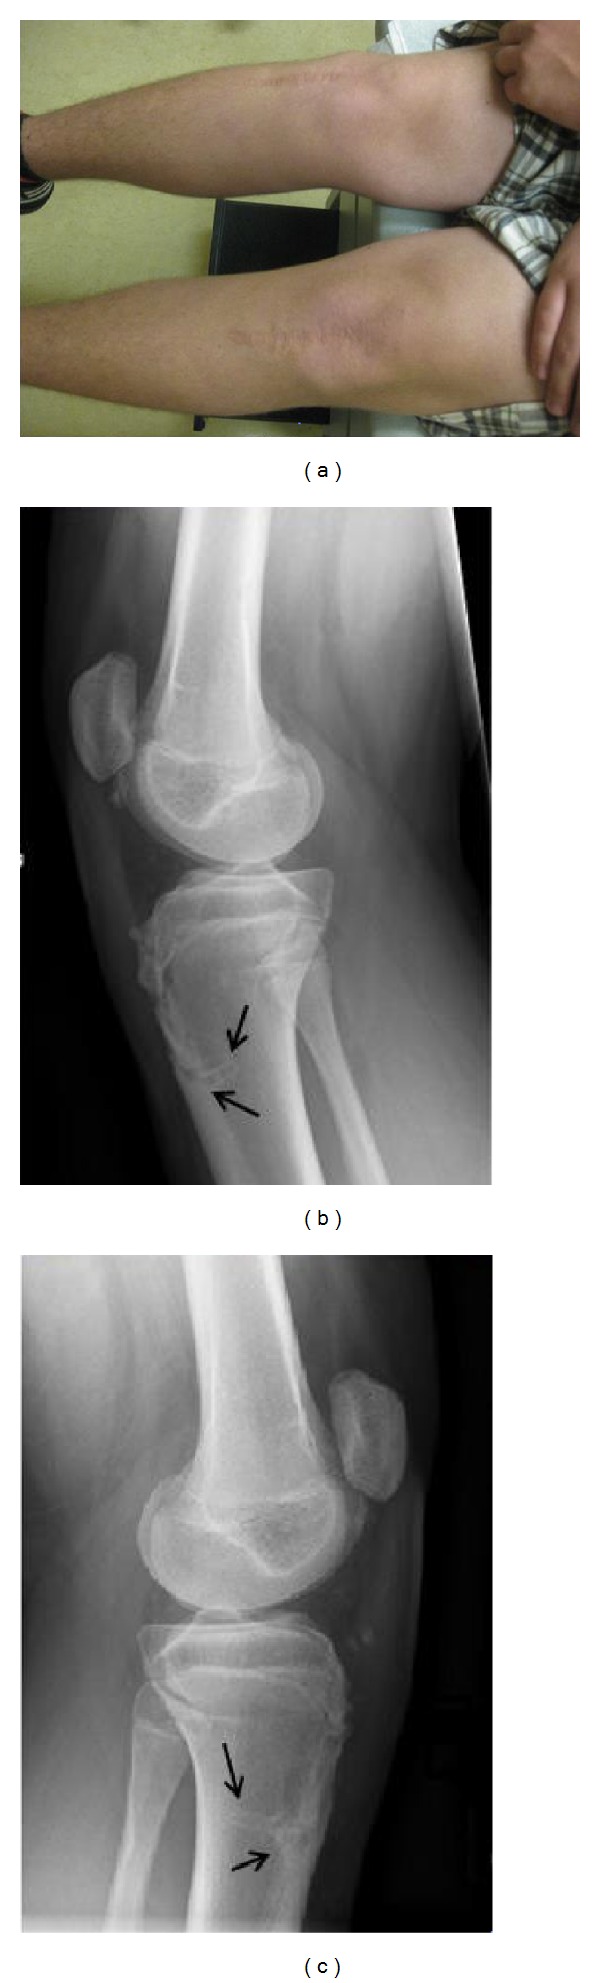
At three-year followup, the patient has full range of active motion and no strength deficits for knee extension. Lateral radiographs show irregularity in the area of patellar tendon repair, sites of suture anchor placement (arrows), and maintenance of posterior tibial slope without any growth disturbances.
